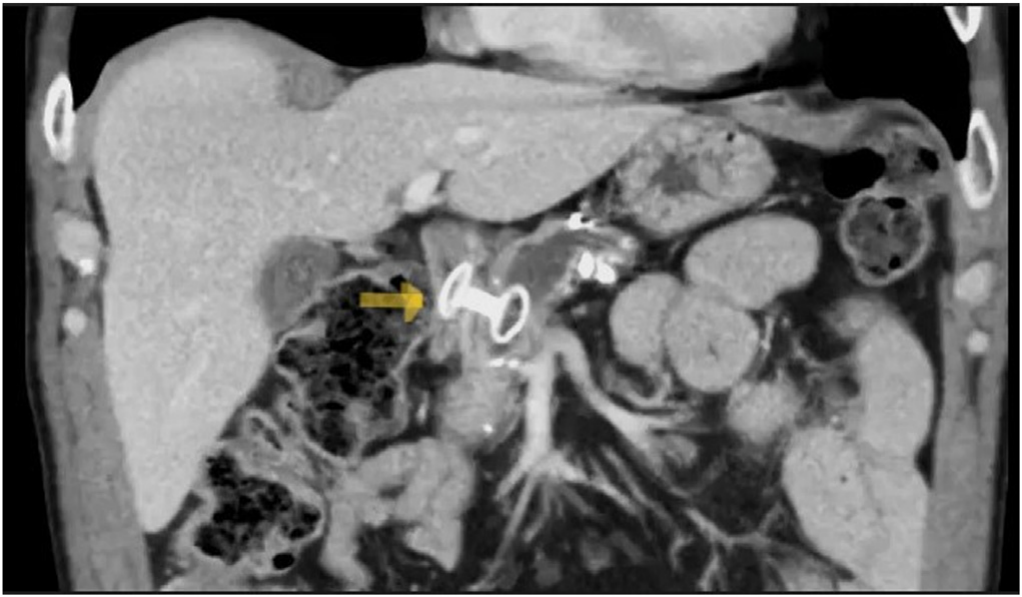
CT image of a lumen-apposing metal stent at the pancreaticoduodenostomy (*yellow arrow*).
